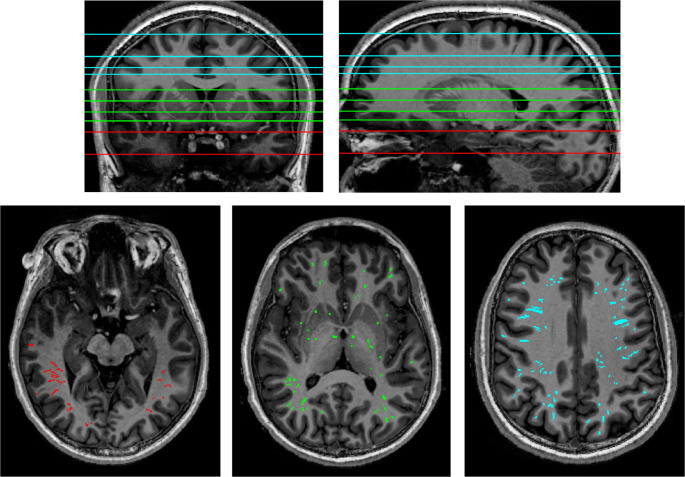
T1-weighted MRI demonstrating the sparse segmentation strategy. Ten Axial slices from each image were selected for manual segmentation (Top-Left: coronal slice; Top-Right: sagittal slice). Red bands indicate the two axial slices at the brainstem (BS) level. Green bands indicate the four axial slices selected at the basal ganglia (BG) level. Cyan bands indicate the four axial slices selected at the centrum semiovale (CS) level. Bottom: axial slices with MRI-PVS labelled
